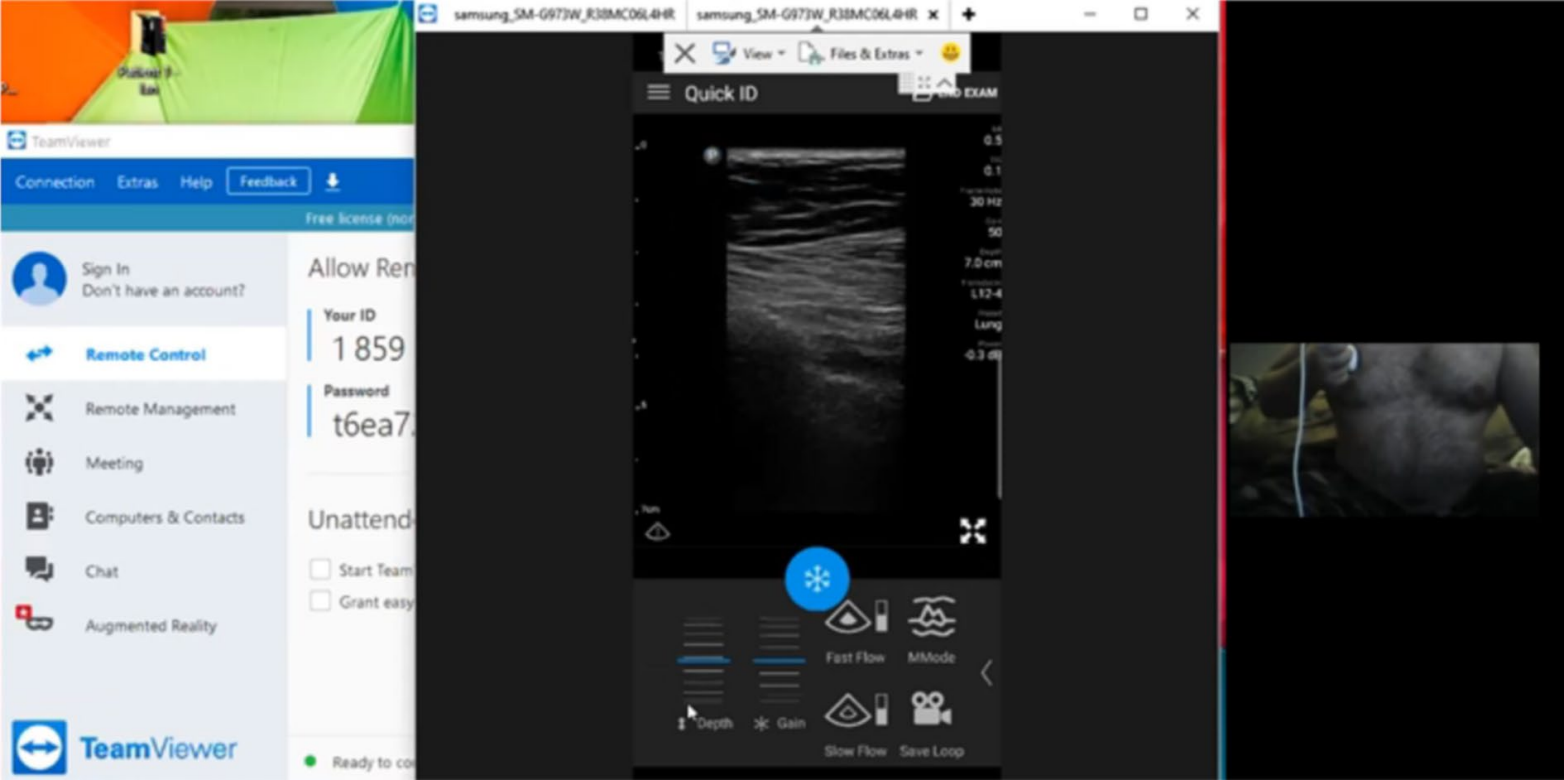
First follow-up Self-Performed Lung Ultrasound Study of 32-year-old patient 2 weeks after COVID-19 diagnosis. Screenshot of the Remote Mentors Computer Screen that shows the patient imaging himself to the right of the screen and the resultant ultrasound image showing the “batwing” sign to the left. As lung ultrasound is a dynamic real-time examination hard to capture in any single image, readers are strongly encouraged to view the accompanying supplemental videos of the entire examination online to gain a true appreciation of the technique
